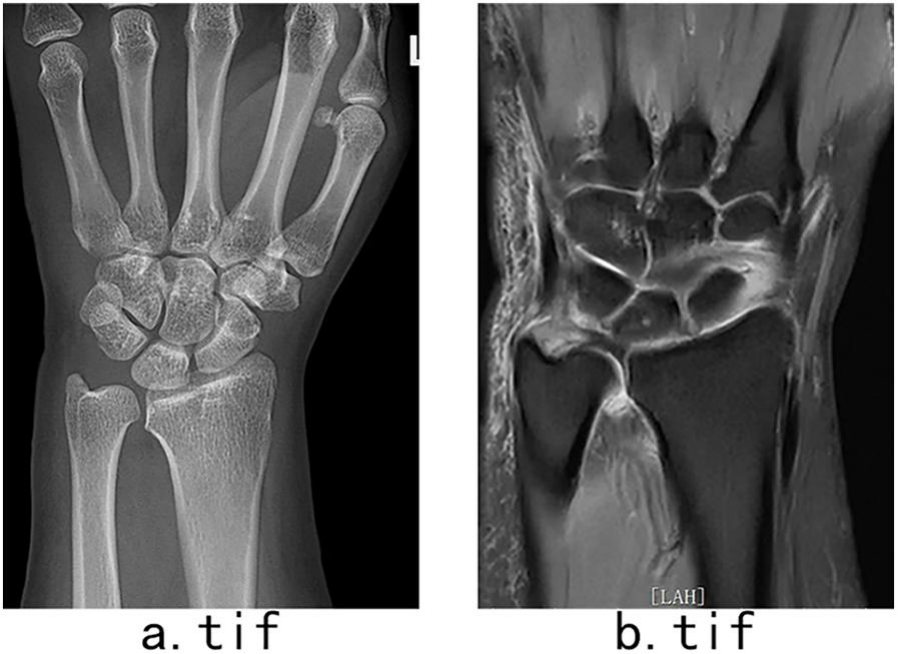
(a) Anterior-posterior X-ray radiograph showed no ulnar-positive variance and slight ulnar chondromalacia. (b) Coronal magnetic resonance radiograph showing perforation of the triangular disk.
